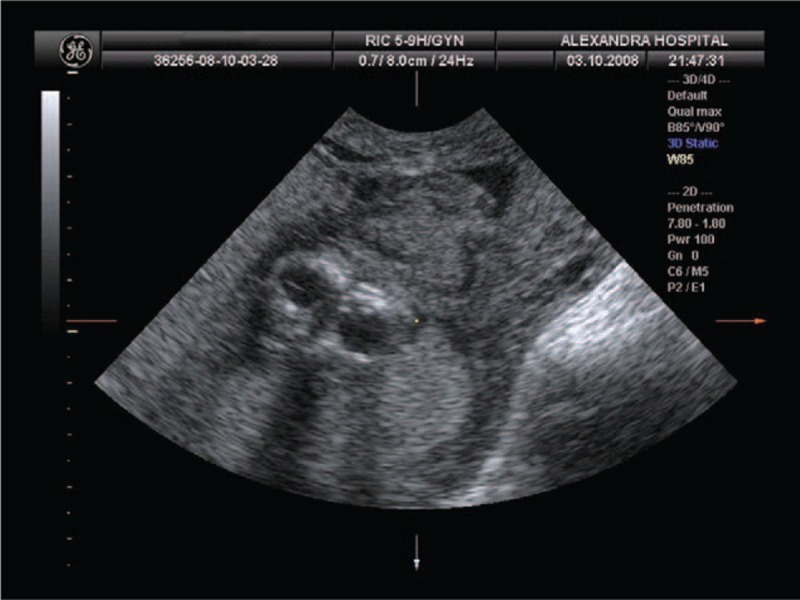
Second trimester ultrasonographic image of an anencephalic fetus.
